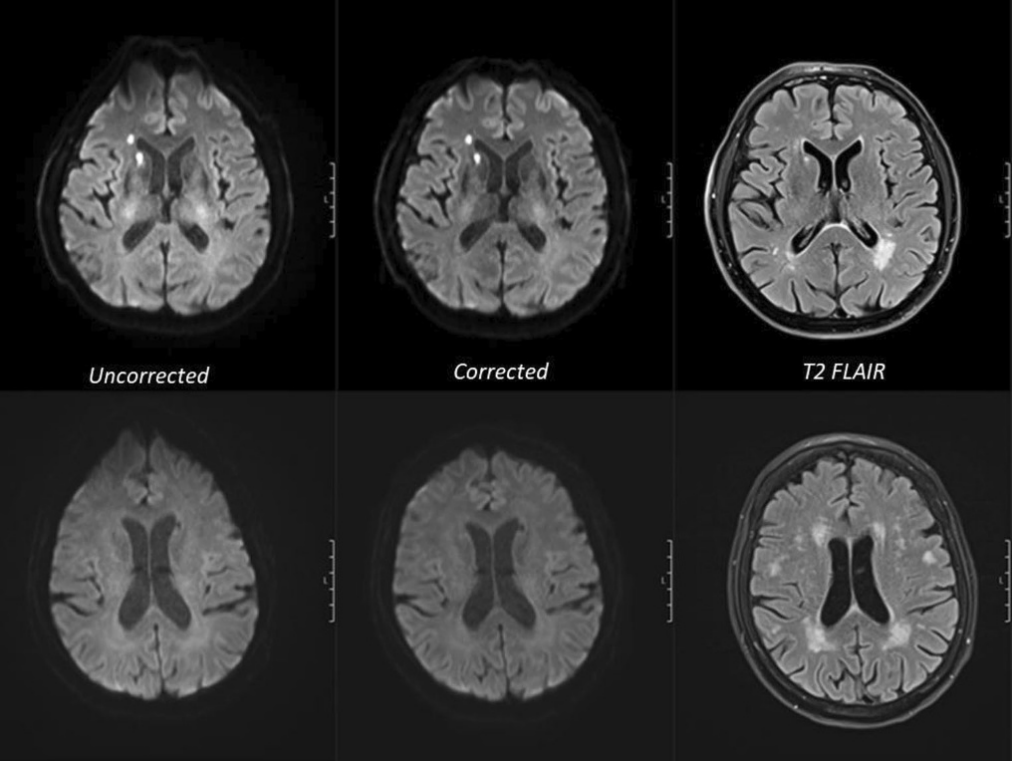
Examples axial MRI scans of two different patients (*upper and lower rows*): corrected EPI diffusion demonstrates a clear reduction of geometric distortion of the frontal lobe in both cases. Matched T2 FLAIR images are provided for comparison
